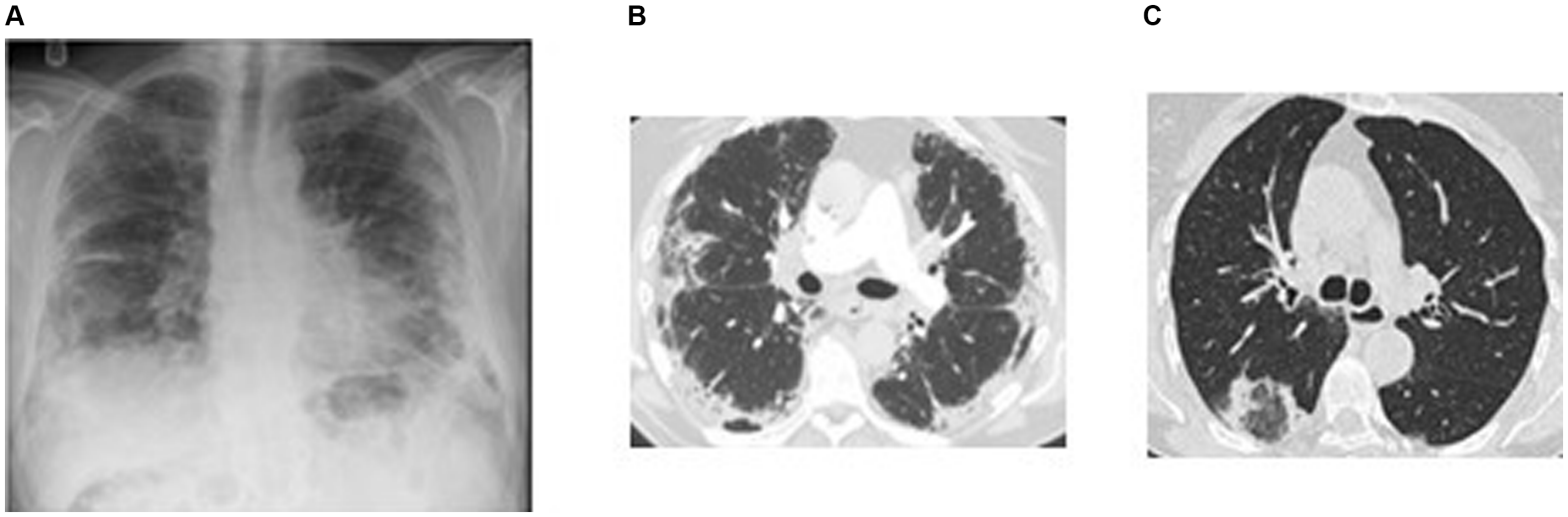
Imaging in chronic eosinophilic pneumonia. (A) Plain chest radiograph in a subject with chronic eosinophilic pneumonia showing peripheral air-space opacification in the mid and lower zones. (B) Chest HRCT scan of the same subject showing peripheral sub-pleural consolidation and ground glass changes with sparing of the central areas. This radiographic feature is typical for eosinophilic pneumonia. (C) Chest HRCT scan in a different subject showing consolidation encircling an area of ground glass opacification resembling a reversed halo (attol sign). This feature is seen in chronic eosinophilic pneumonia but also in a number of other pathologies including COP, sarcoidosis, vasculitis, and infection.
